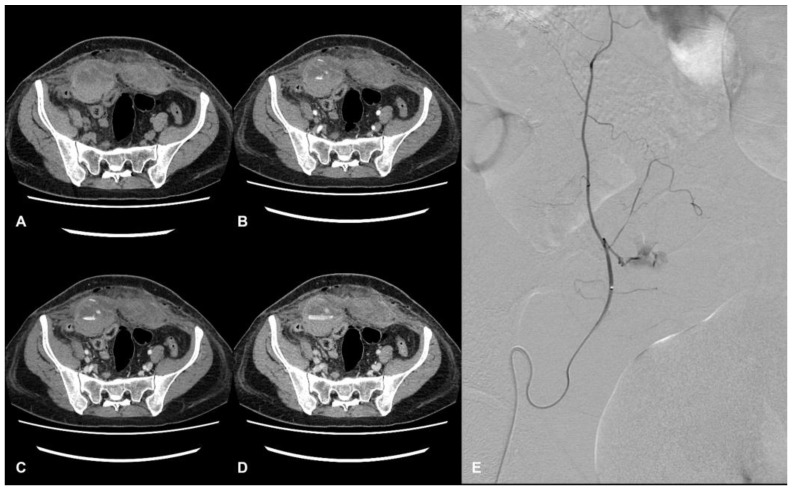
CT and DSA images of a hospitalized patient with COVID-19. Evidence of a large hematoma within rectus abdominis below the arcuate line in axial CT images. The pre-contrast phase shows the extension and location of the hematoma, showing a fluid level (hematocrit effect) (A). The contrast-enhanced acquisition at the arterial (B), portal (C), and delayed phase (D) show active bleeding within the hematoma. Angiography shows a contrast blush from branches of the right inferior epigastric artery (E).
